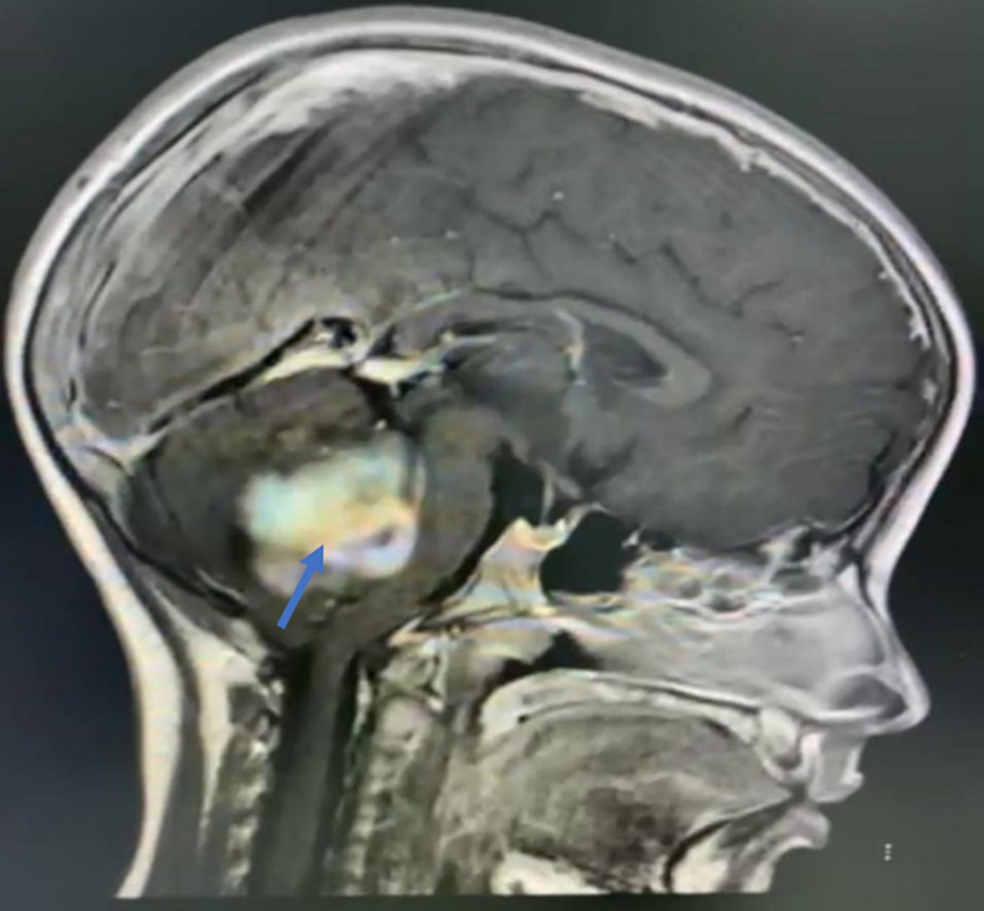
Preoperative image - MRI brain sagittal cut shows obstructive hydrocephalus
